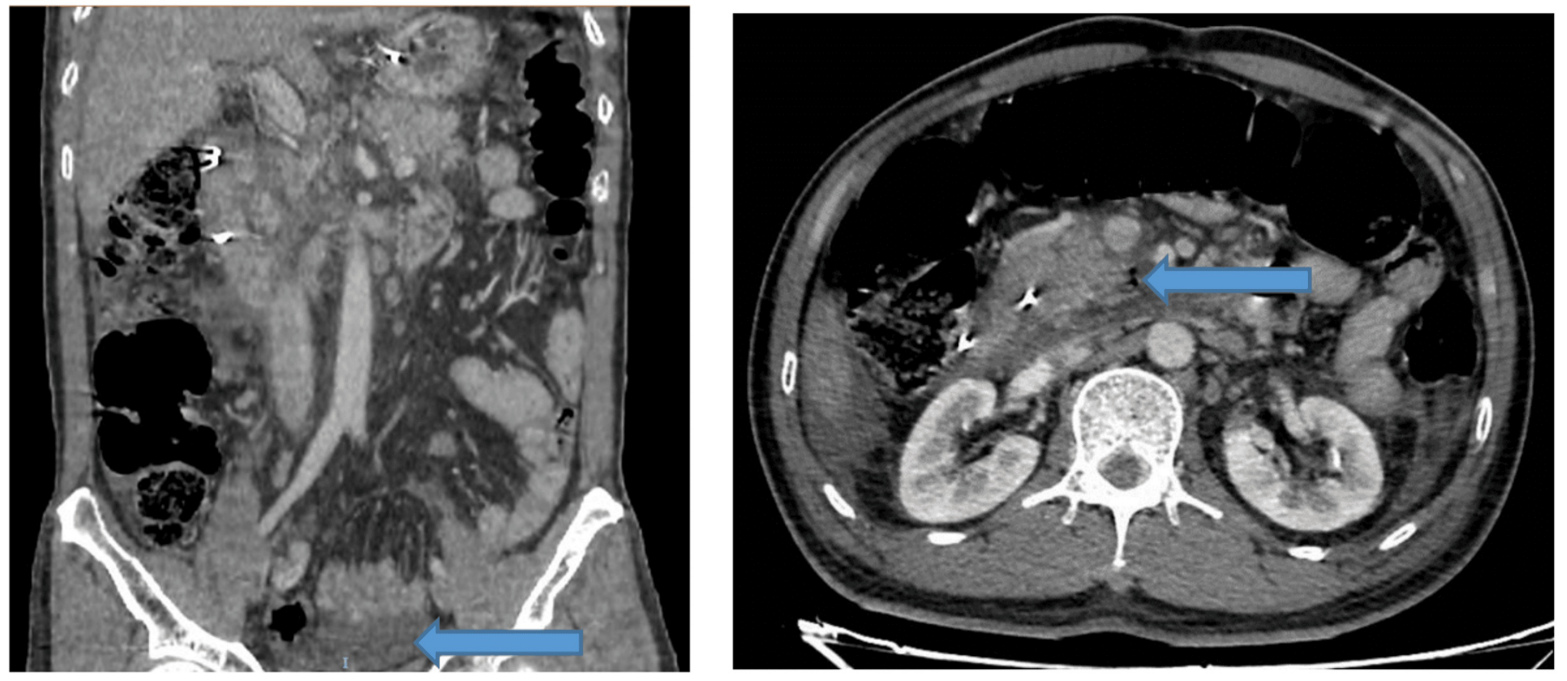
Computed tomography showed retroperitoneal free air in the peripancreatic and periduodenal regions and moderate ascites and mesenteric fat stranding.
